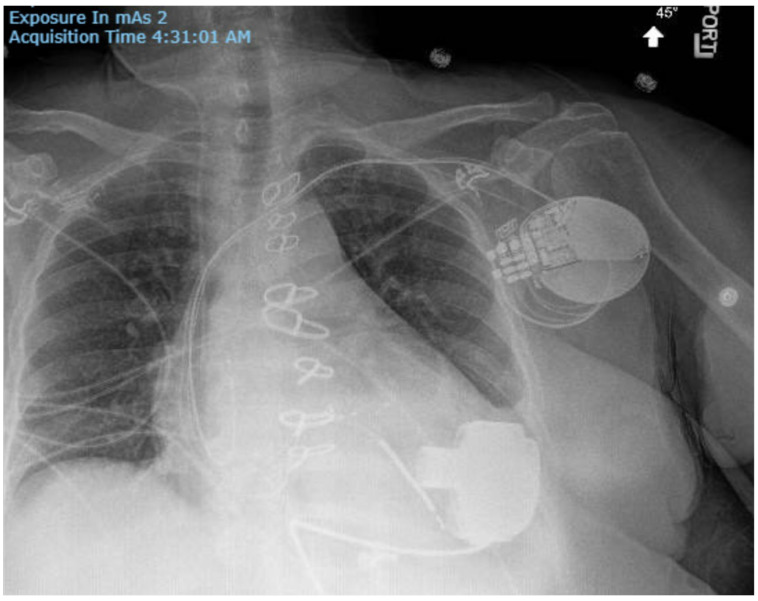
Chest X-ray. Posterior–anterior view. Patient with a transvenous cardiac resynchronization therapy-defibrillator and a left ventricular assist device.
